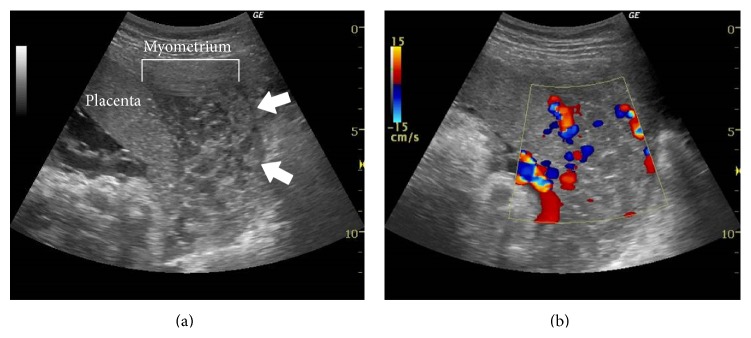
Ultrasonography demonstrated tubular echolucent spaces throughout the myometrium (a), and color Doppler showed blood flow within some of the cystic lesions (b) at 27 weeks of gestation.
